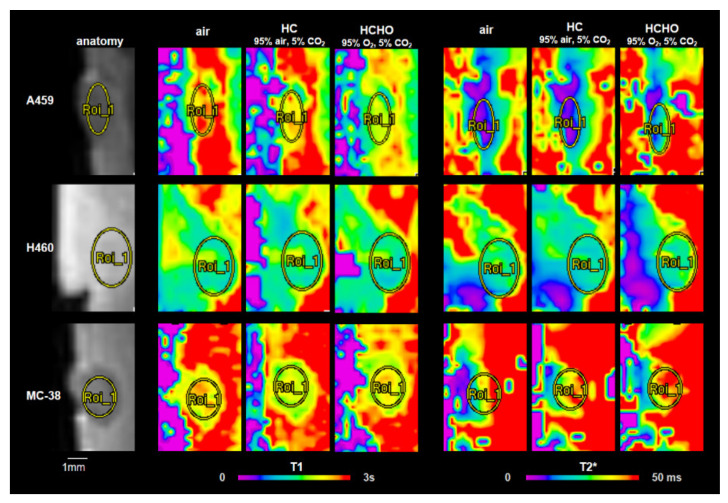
Representative MRI images of A549, H460, and MC-38 cell grafts grown on the CAM in ovo. Grafts are outlined on T1w anatomical reference images (left column) and quantitative color-coded T1 and T2* maps obtained while the graft was exposed to medical air, hypercapnia (HC), and hypercapnic-hyperoxia (HCHO). In H460 and MC-38 grafts, larger T1 and T2* were located in the graft center, revealing a distinct spatial distribution of T1 and T2* within the graft and response to gas challenge.
